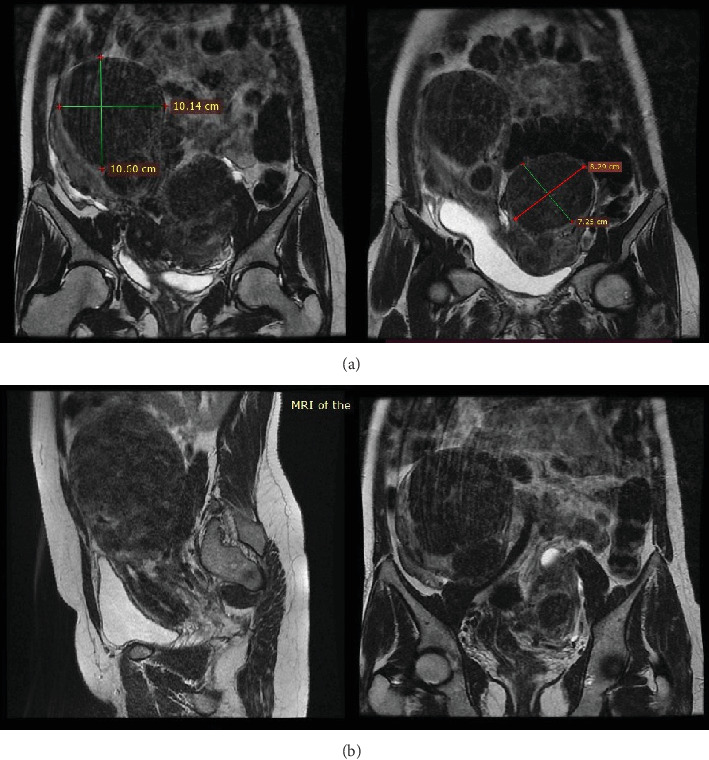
(a, b) MRI showing the initial finding of 10.14 × 10.6 cm mass in the right horn and 7.25 × 8.29 cm in the left horn. (b) Two distinct cervices with an upper vaginal septum.
